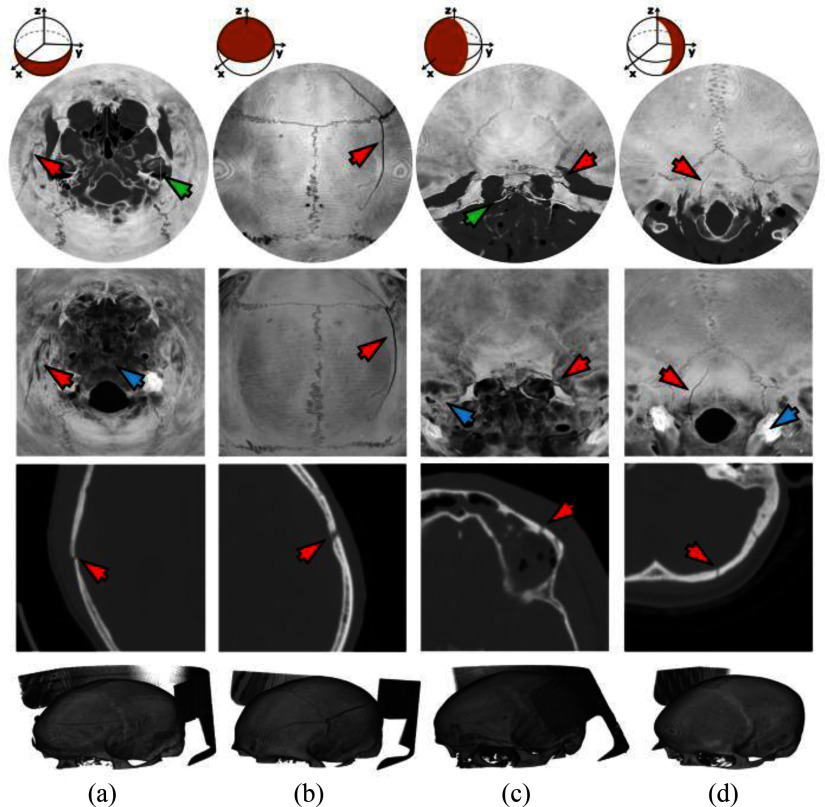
Comparative visualization of skull fractures in four patients, displaying different fractures, at their corresponding skull quadrant region: (a) Patient 107-Lower, (b) Patient 13-Upper, (c) Patient 241-Frontal, and (d) Patient 205-Occipital hemisphere projection. First row: disk harmonic mapping MIP, second row: AEM MIP [24], third row: magnified regions of axial CT slices, and fourth row: DICOM 3D reconstructions. The arrows indicate fractures (red), imaging artifacts (green), and obscured regions (blue). The fracture of Patient 107 was missed by one radiologists. Patients 13 and 241 were correctly diagnosed by all three whereas the fracture of Patient 205 was missed by 2 out of 3 radiologists.
